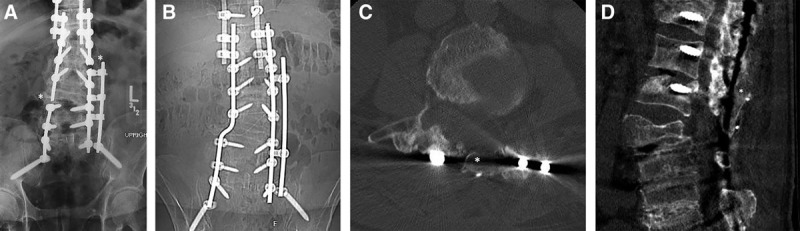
Preoperative and postoperative imaging of a patient undergoing application of vascularized pedicled iliac crest to treat recurrent lumbar and sacral pseudarthrosis. A, Preoperative anteroposterior (AP) lumbar spine x-ray demonstrating bilateral rod fractures (*) with underlying pseudarthroses. B, One year postoperative AP lumbar spine x-ray with revised spinal hardware and faint bone graft over the mid-lumbar spine. C, Postoperative computed tomography (CT) scan, axial cut, at the L3 level showing the cortical edges of the iliac crest bone graft (*). D, Postoperative CT scan, sagittal cut, with the bone graft partially obscured by the hardware artifact (*).
